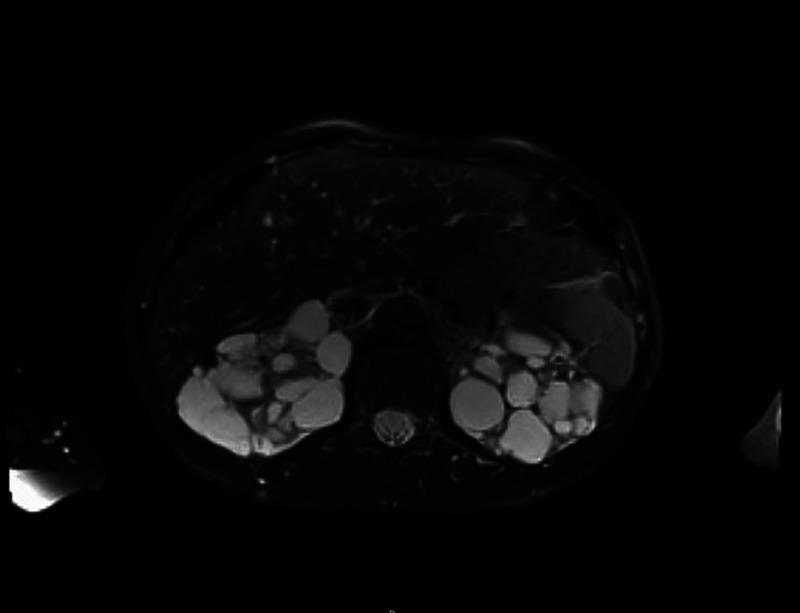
Magnetic resonance imaging 4 weeks post-intervention demonstrating complete resolution of the cyst.
